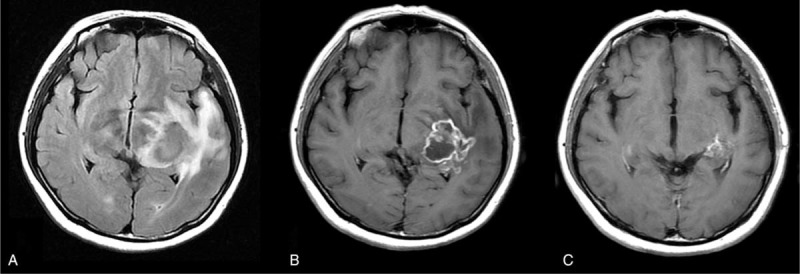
MRI findings in 2020 FLAIR (A) showed a hypointense mass in the left temporal lobe with severe vasogenic edema. Post contrast T1WI (B) showed irregular rim-enhancement. The lesion regressed significantly after rituximab treatment (C).
